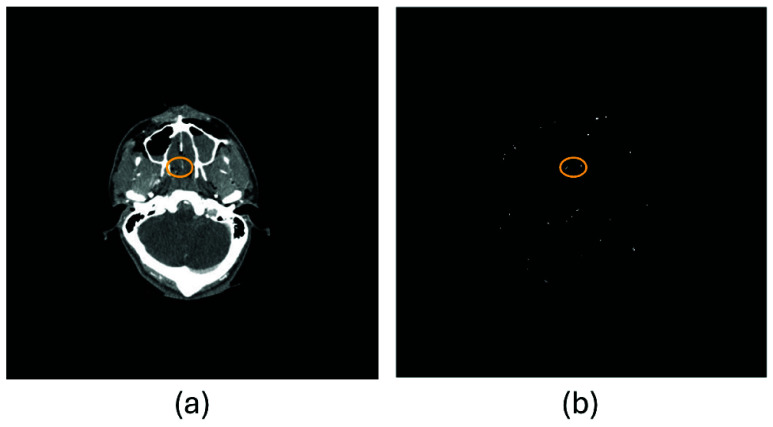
Contrast extravasation detection in a facial trauma case with fracture. (a) Axial CT slice showing a surgeon-identified extravasation focus (circled). (b) Corresponding KGA activation map highlighting candidate regions, where the extravasation region is circled and a positive alert is generated as the confidence score exceeds the detection threshold.
